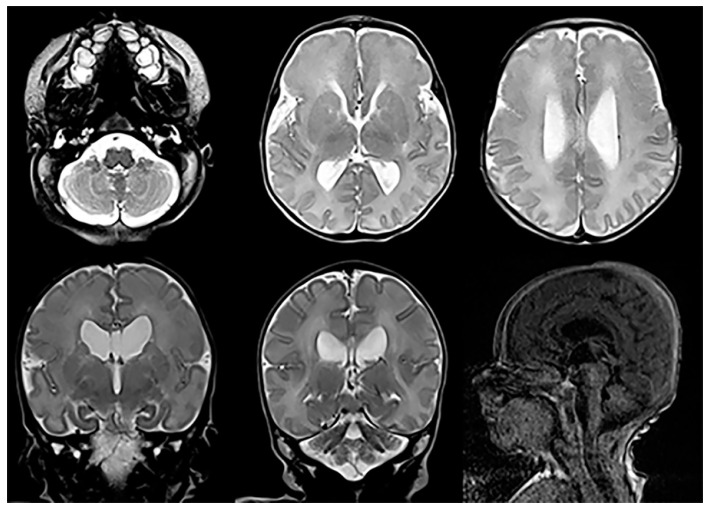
Axial and coronal T2-weighted and sagittal T1-weighted MRIs in a child with confirmed Schinzel-Giedion syndrome reveal mild cerebellar and vermian hypoplasia, a shallow pontine belly, and mild ventriculomegaly.
